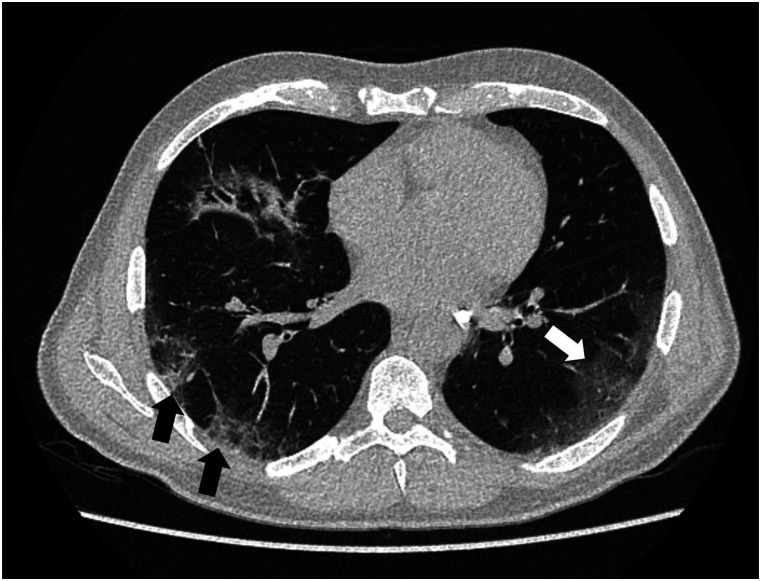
67-year-old male patient with suspected and ultimately confirmed SARS-CoV-2 infection, who presented with fever, dry cough, dyspnea, headaches, and myalgia. Chest CT revealed peripherally accentuated ground-glass opacities (white arrow) and consolidations (black arrows) without pleural effusion. Quality rating: 0 (optimal quality). DLP: 83.4 mGy*cm.
